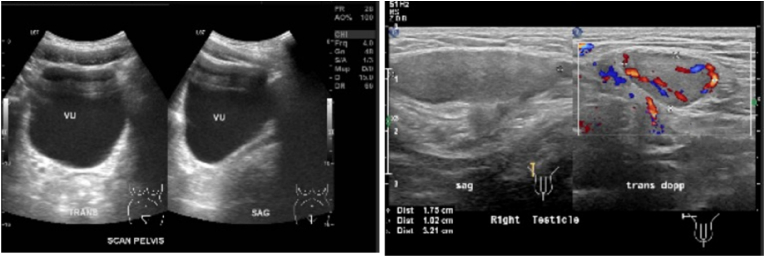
Ultrasonography result: (a) Internal Genitals, (b) Testicular.
